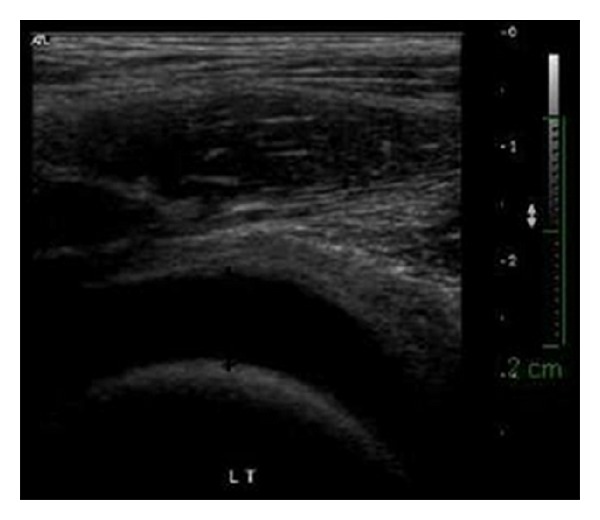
Ultrasound of patient's left hip at the emergency room showing distention of the hip joint due to increased fluid level.
